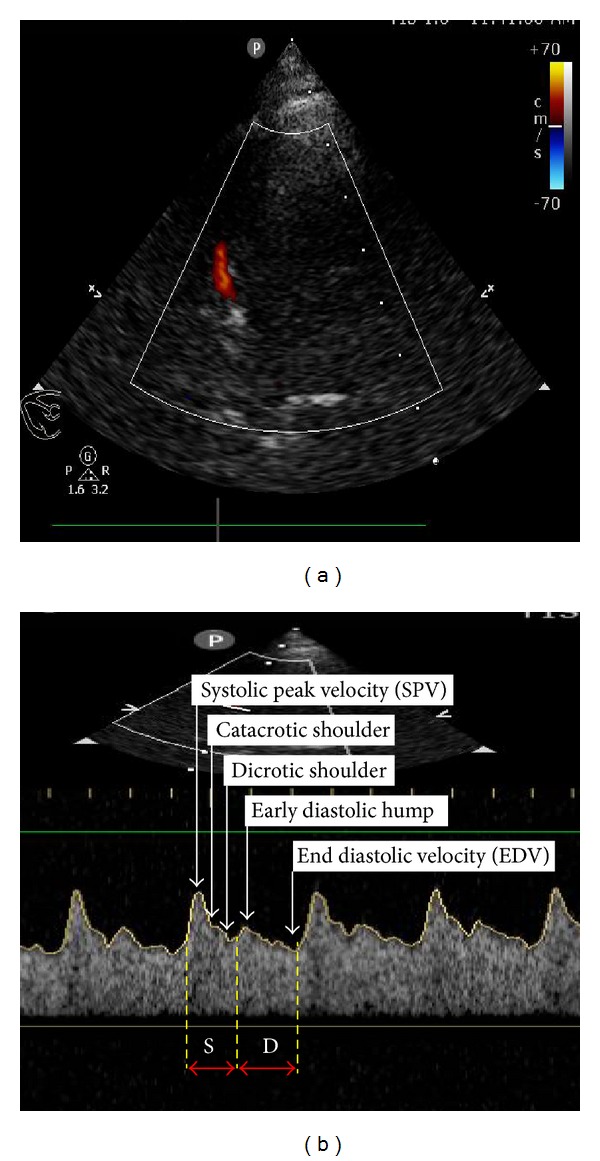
Transcranial color coded duplex (TCCD): (a) middle cerebral artery (MCA) color Doppler and (b) MCA pulsed wave Doppler. S: systole, D: diastole.
